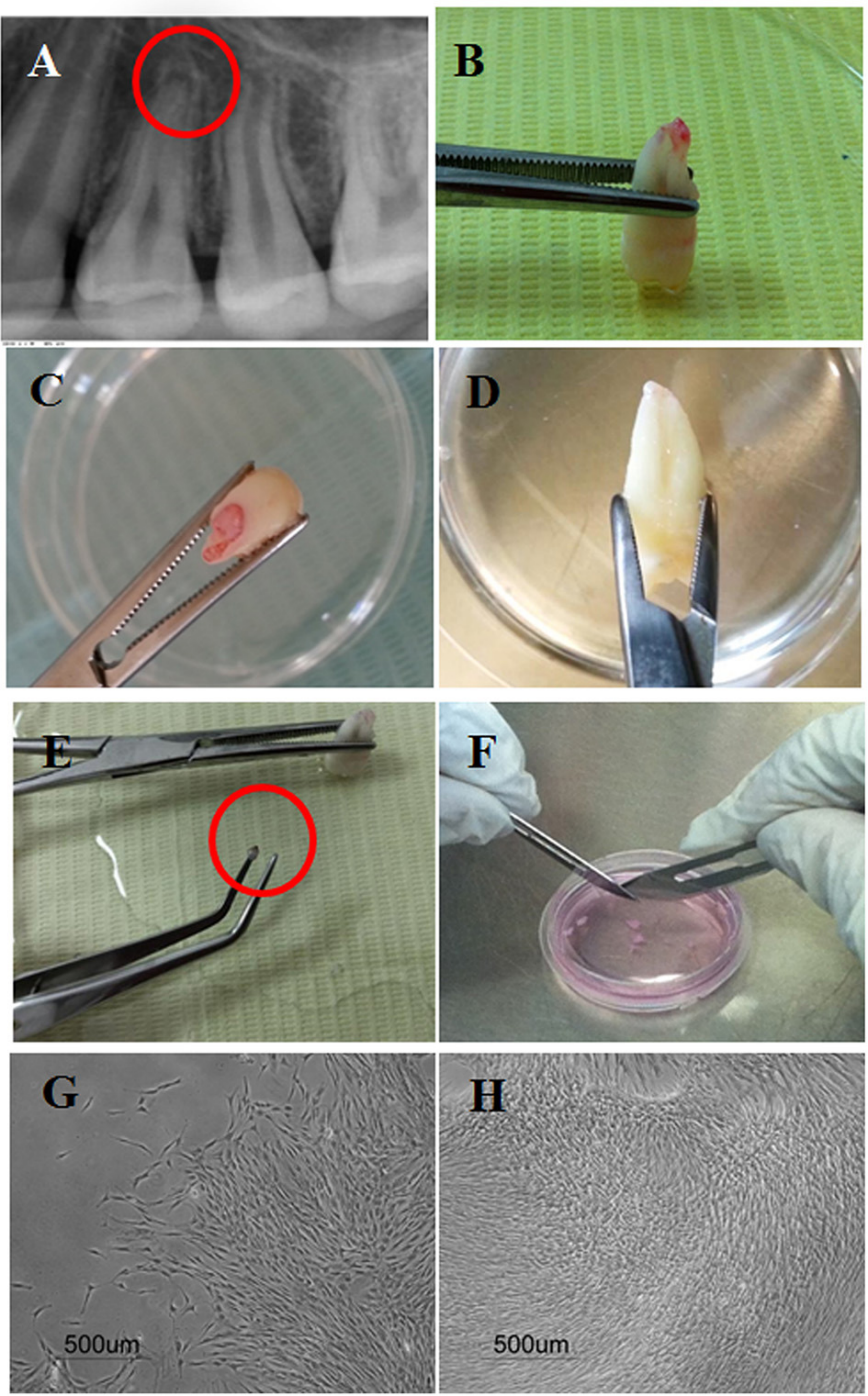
Isolation of stem cells from the apical papilla showing: (A) Periapical radiographs of the apical papillae related to the apex of a human premolar; (B–D) Extracted premolars with intact apical papillae. (E) Apical papilla separated gently using tweezers; (F) Scalpel dissection of apical papilla tissue in a cell culture dish with culture medium. (G,H) Cultured human SCAP at 4x after primary culture at 8 and 21 days, respectively.
